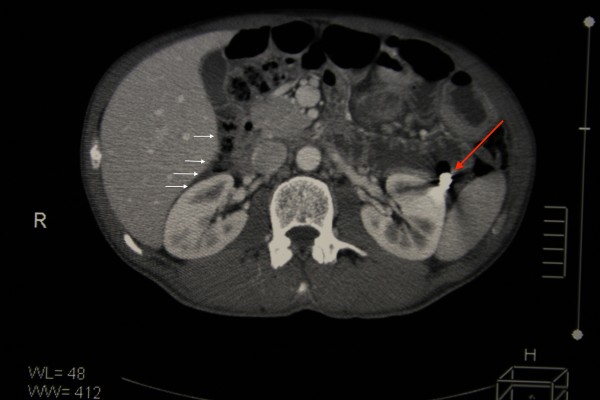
Computed tomography showing the capsule endoscope impacted in the ileum (red arrow) with extensive small bowel dilation along with free peritoneal air (white arrows).
